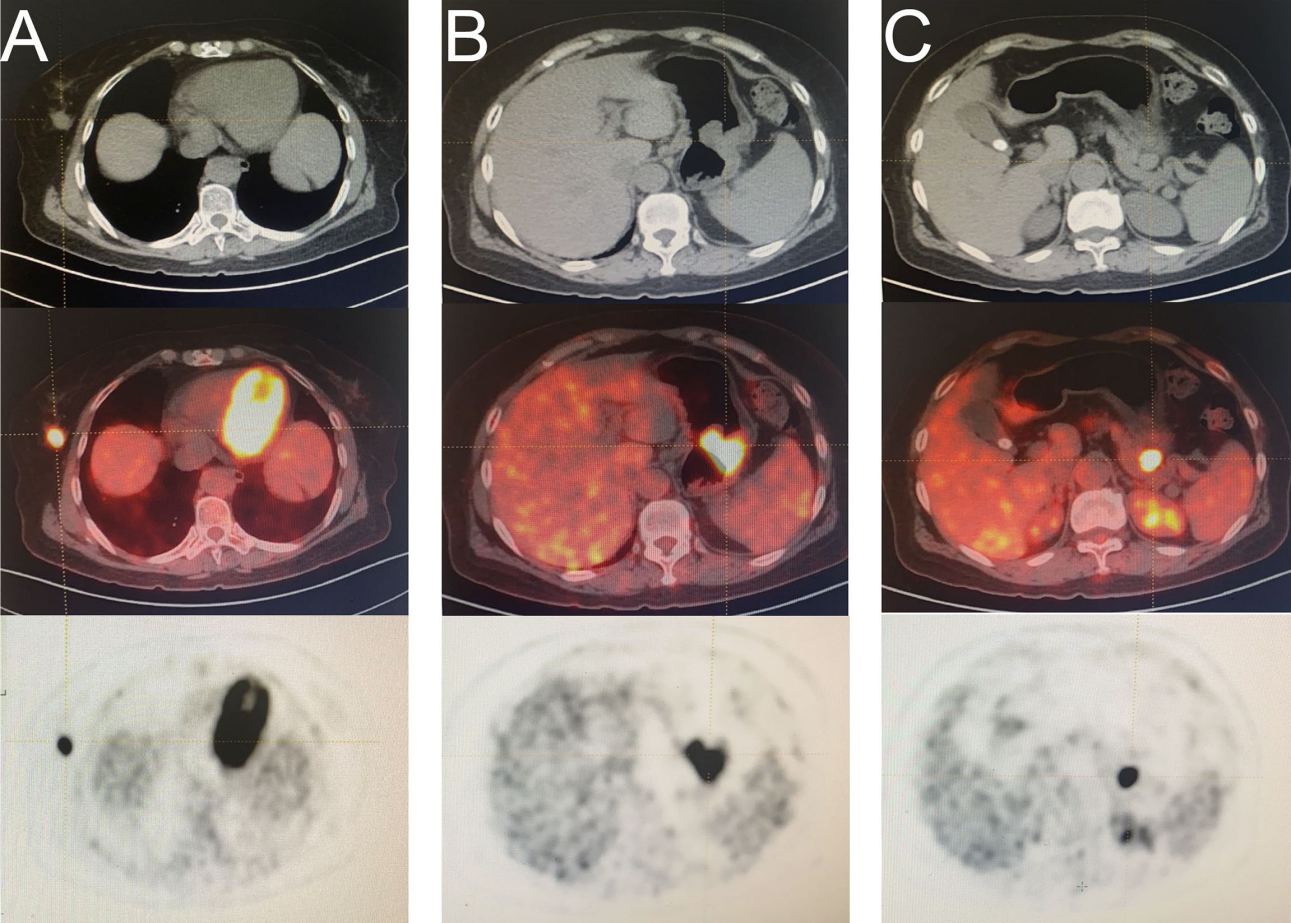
A F18-fluorodeoxyglucose (FDG) positron emission tomography computed tomography (PET-CT) scan was conducted. (A) mass in right breast (B) mass in the greater curvature of the stomach (C) mass in the space between the stomach and pancreas.
